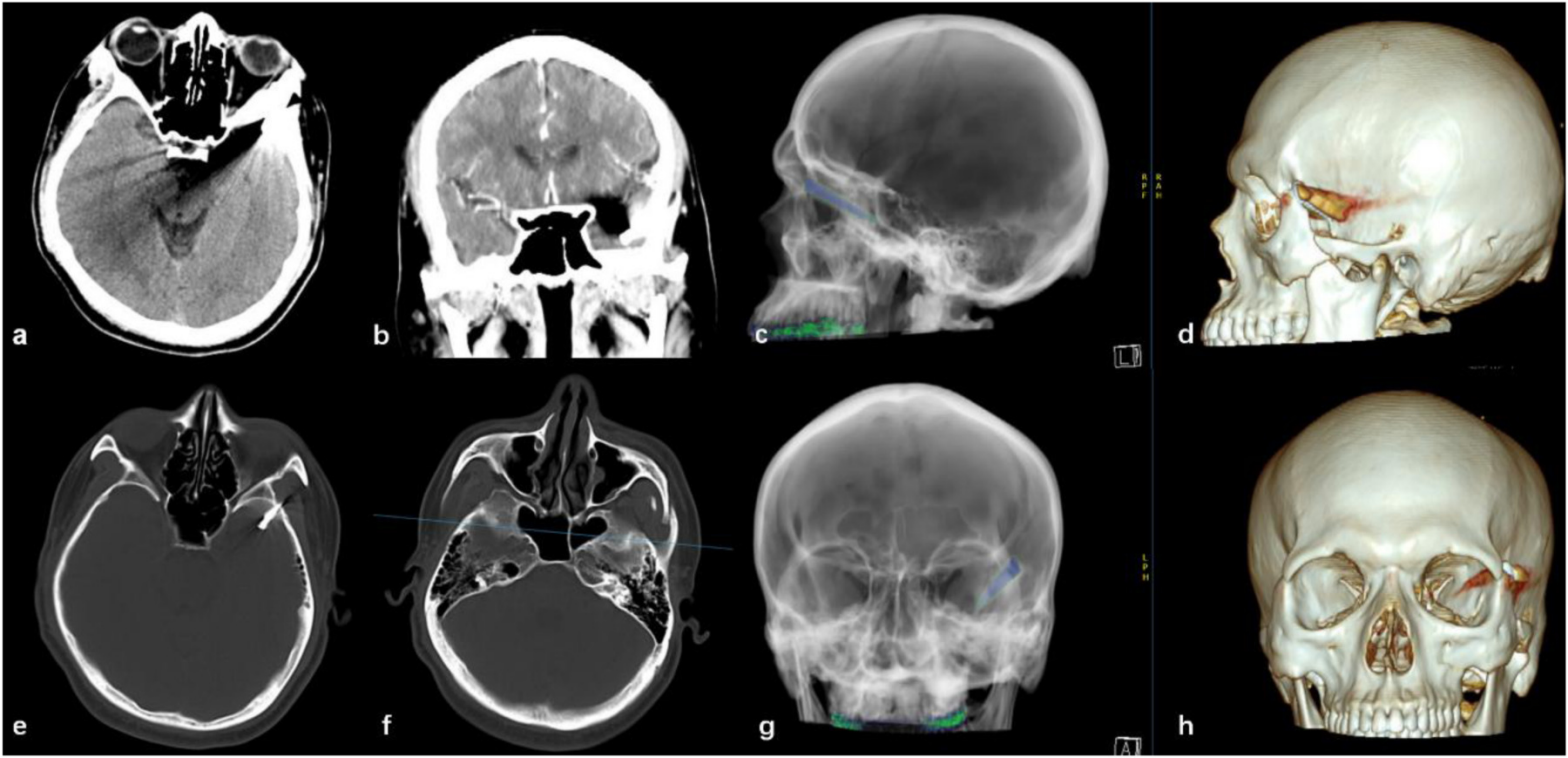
(a) Axial CT scan showing a foreign body penetrating the lateral orbital wall into the cranial cavity; (b) enhanced coronal CT showing the penetrating foreign body located in the left temporal lobe, at a distance from the sylvian fissure vessels; (e,f) axial CT bone window images showing a conical, elongated foreign body penetrating the temporal bone into the cranial cavity, with a fracture at the left zygomatic bone penetration site; (c,d,g,h) three-dimensional reconstruction of the intracranial foreign body and skull, showing anterior and lateral relationships.
